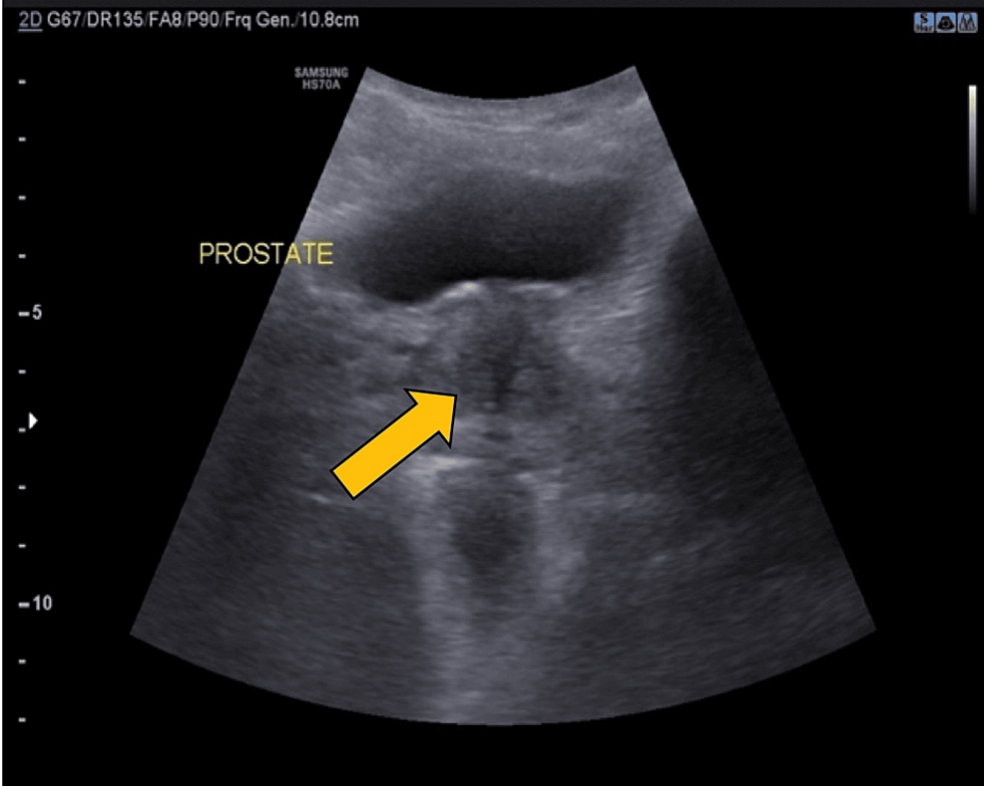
The prostate appearing heterogenous on ultrasonography, suggesting prostatitis
